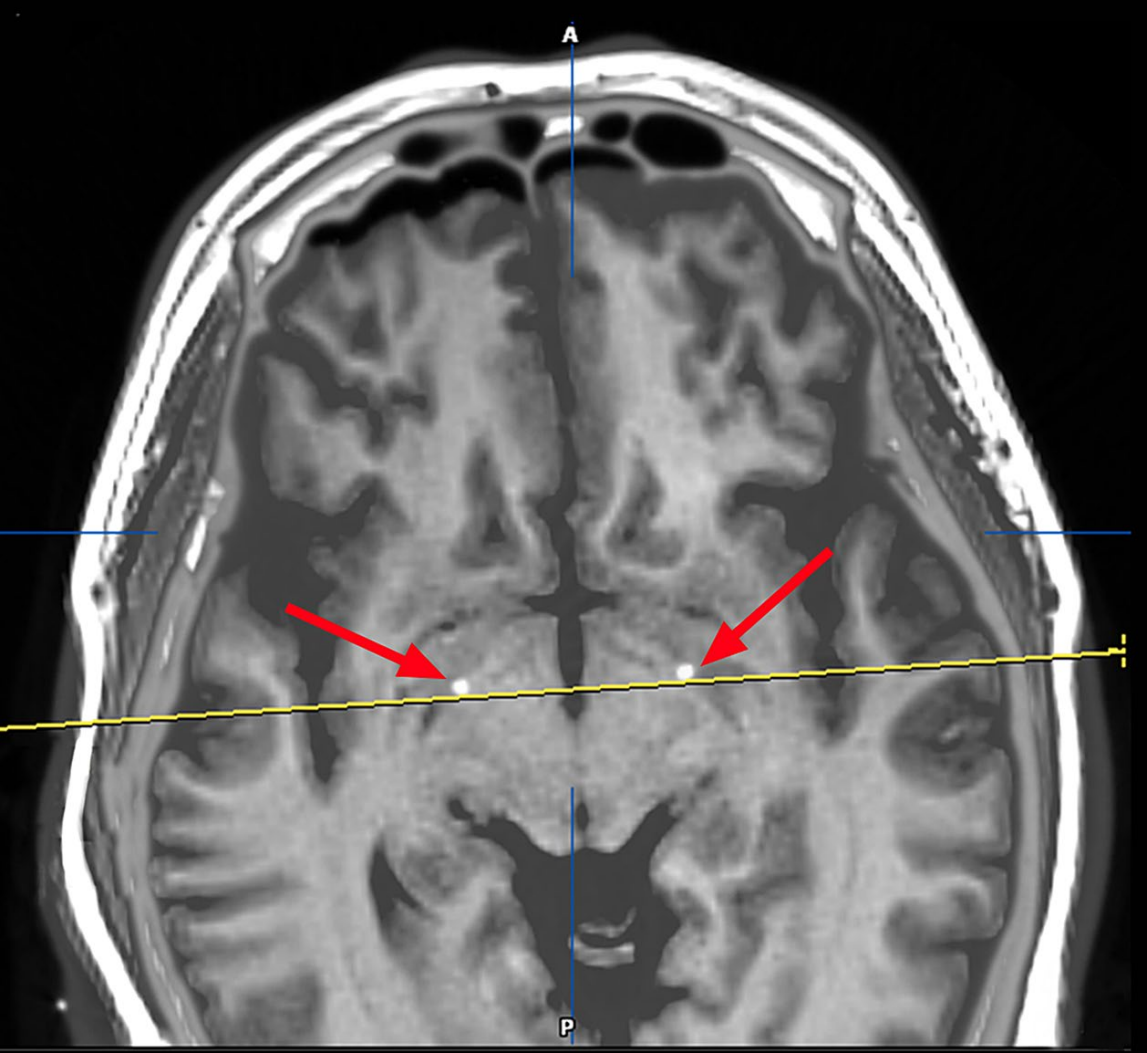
Image after fusion of preoperative MR plan and postoperative CT and it is a postoperative image fusion, suggesting that the stimulation electrode is precisely located in the GPI nucleus.
